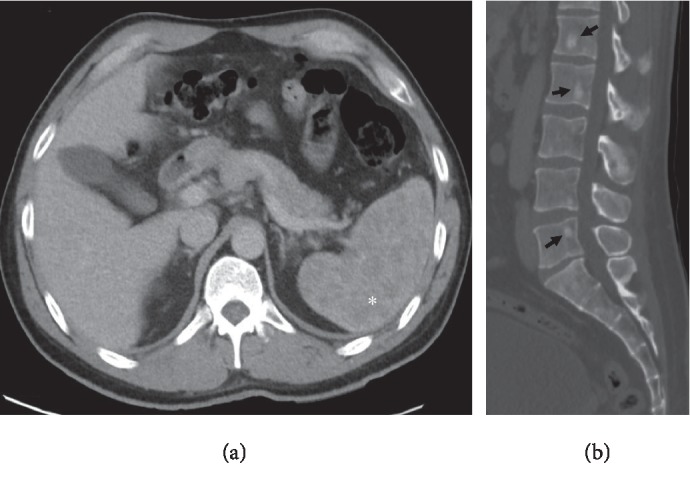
Contrast-enhanced CT of the abdomen pelvis demonstrates an enlarged and heterogeneous spleen (asterisk (a)) and multiple nonspecific sclerotic osseous lesions within the lumbar spine and sacrum (arrows (b)).
